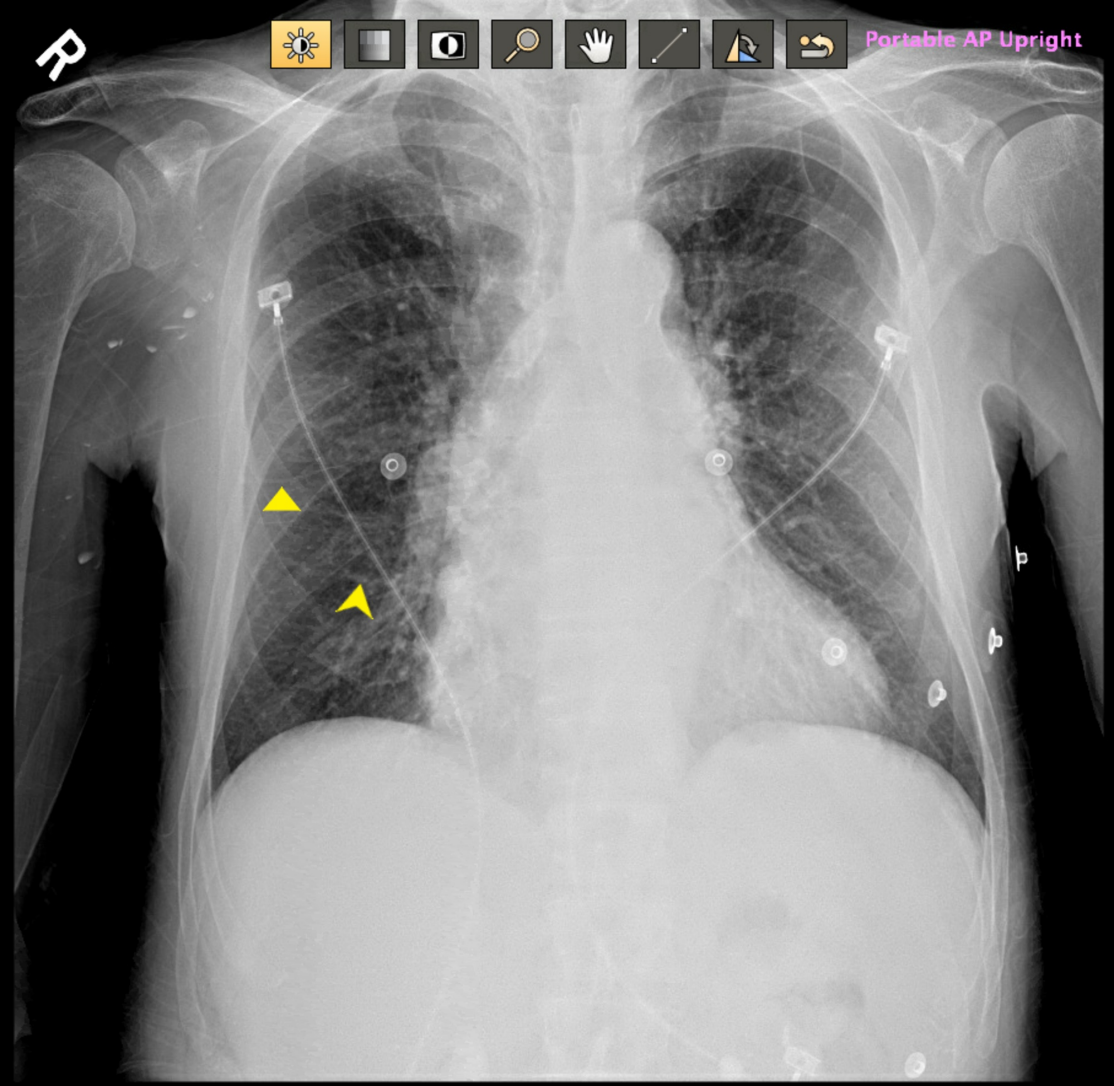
Chest x-ray revealing mild pulmonary edema Yellow arrows marking Kerley B lines suggestive of pulmonary edema
